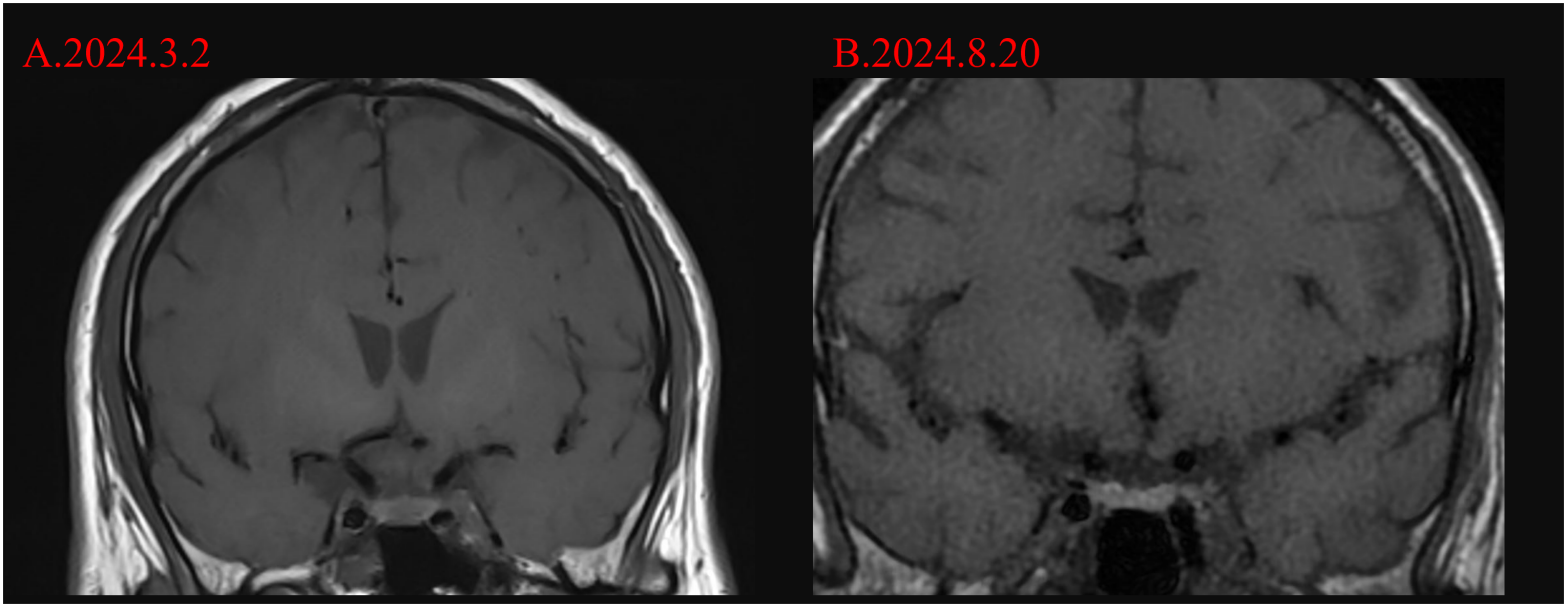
MRI changes of the pituitary gland before and after the occurrence of adrenal crisis in the patient. (A) shows the T2-weighted image of the pituitary gland of the patient before the occurrence of adrenal crisis (March 2, 2024), at this time the patient did not show any manifestations of adrenal cortical insufficiency such as hyponatremia; (B) shows the T2-weighted image of the pituitary gland of the patient on the third day after the occurrence of adrenal crisis (August 20, 2024), at this time the pituitary gland was only slightly thinner, and no obvious abnormalities were observed.
